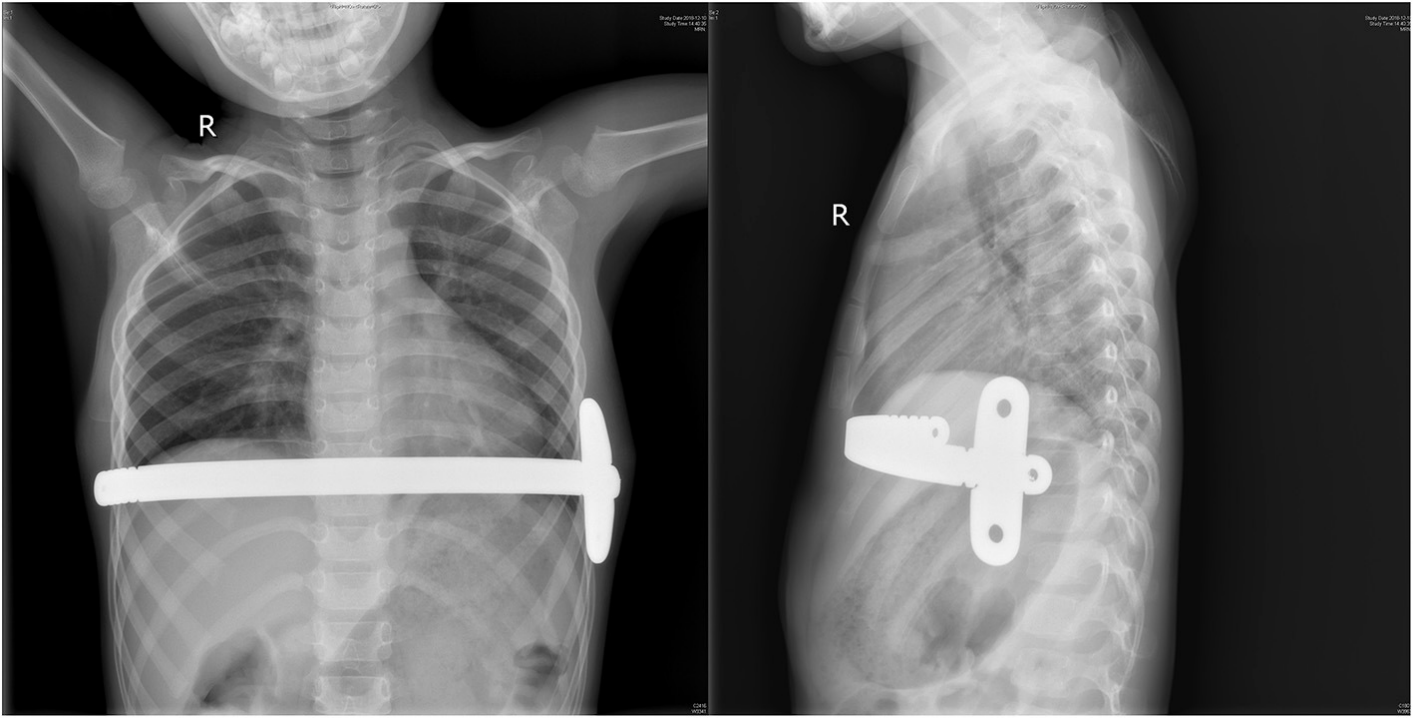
The chest radiograph showed that bar placement and fixation 3 days after surgery.
